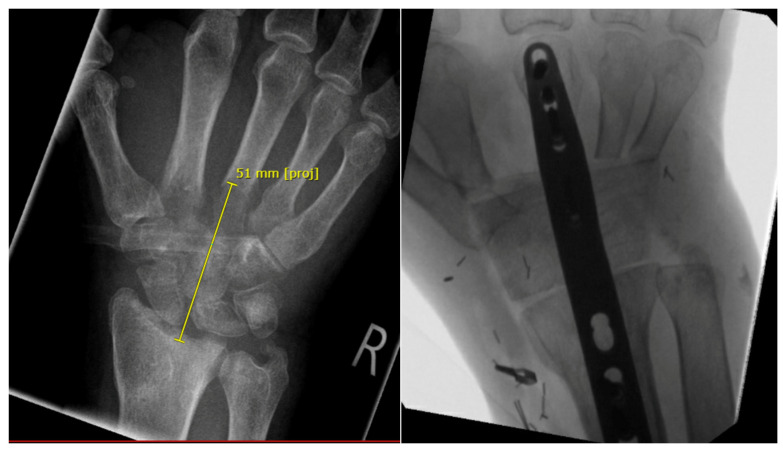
Preoperative X-ray (left) with osteitis of the carpal bones and the proximal metacarpal bones. Intra-operative X-ray with reconstruction of the space between the distal radius and the middle hand with a free vascularized iliac crest (right).
